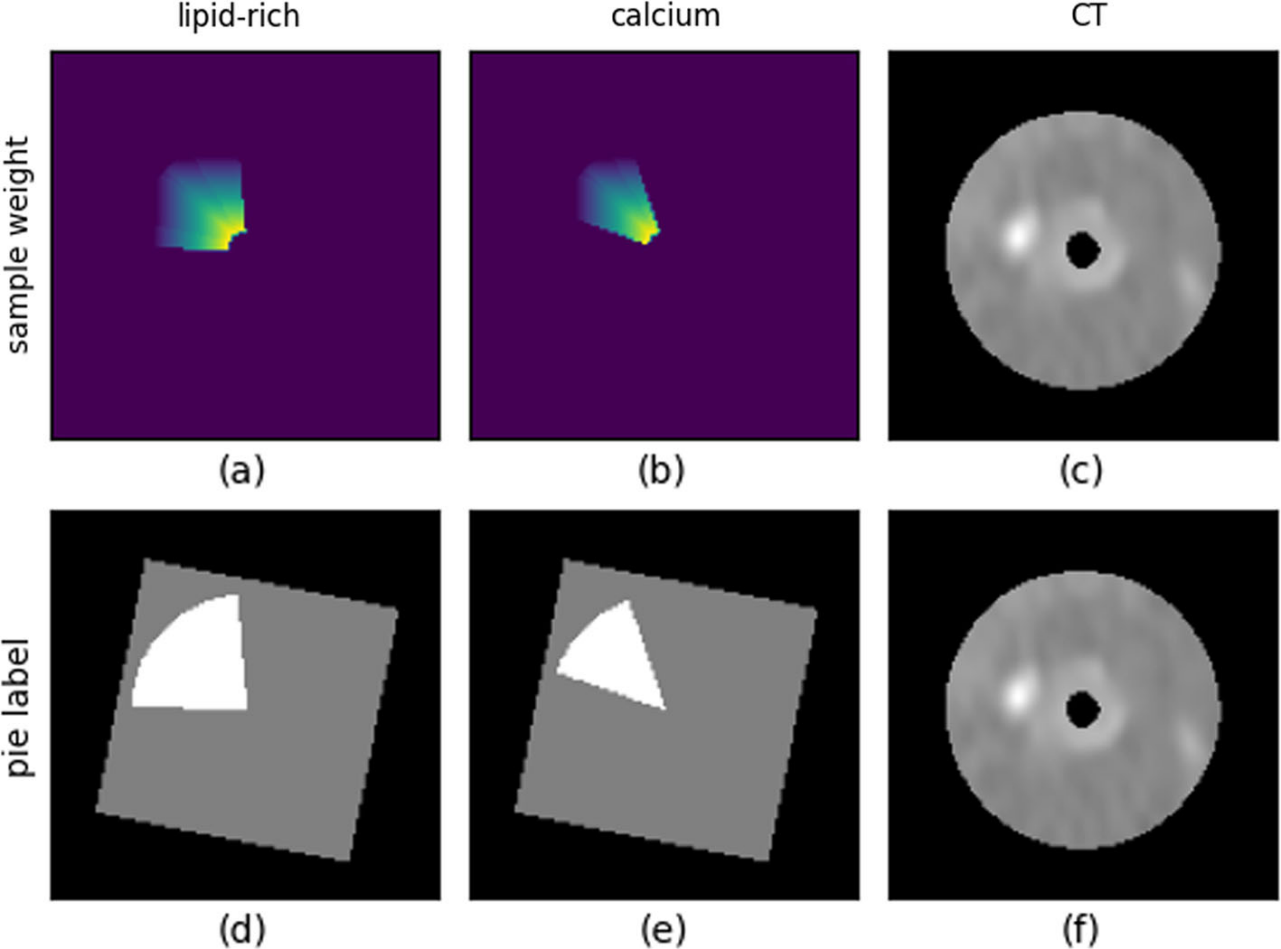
Binary labels and sample weights used for 2.5D Dense U-Net. a, b: pixel-wise gradient sample weights for lipid-rich plaques and calcified plaques; d, e: binary pie-shaped labels for lipid-rich and calcified plaques; c, f cross-sectional CT image
